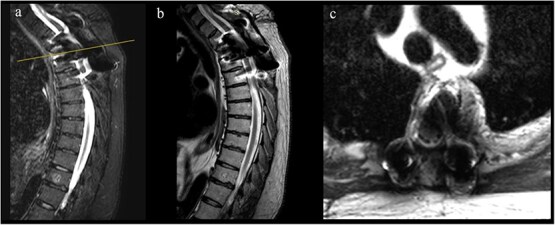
1-year follow-up MRI. (a, b) Sagittal T2-weighted images showing decompression of the spinal cord at T3 with resolution of the epidural component and susceptibility artifact from arthrodesis material. (c) Axial T2-weighted image confirming adequate canal decompression with artifact related to instrumentation.
